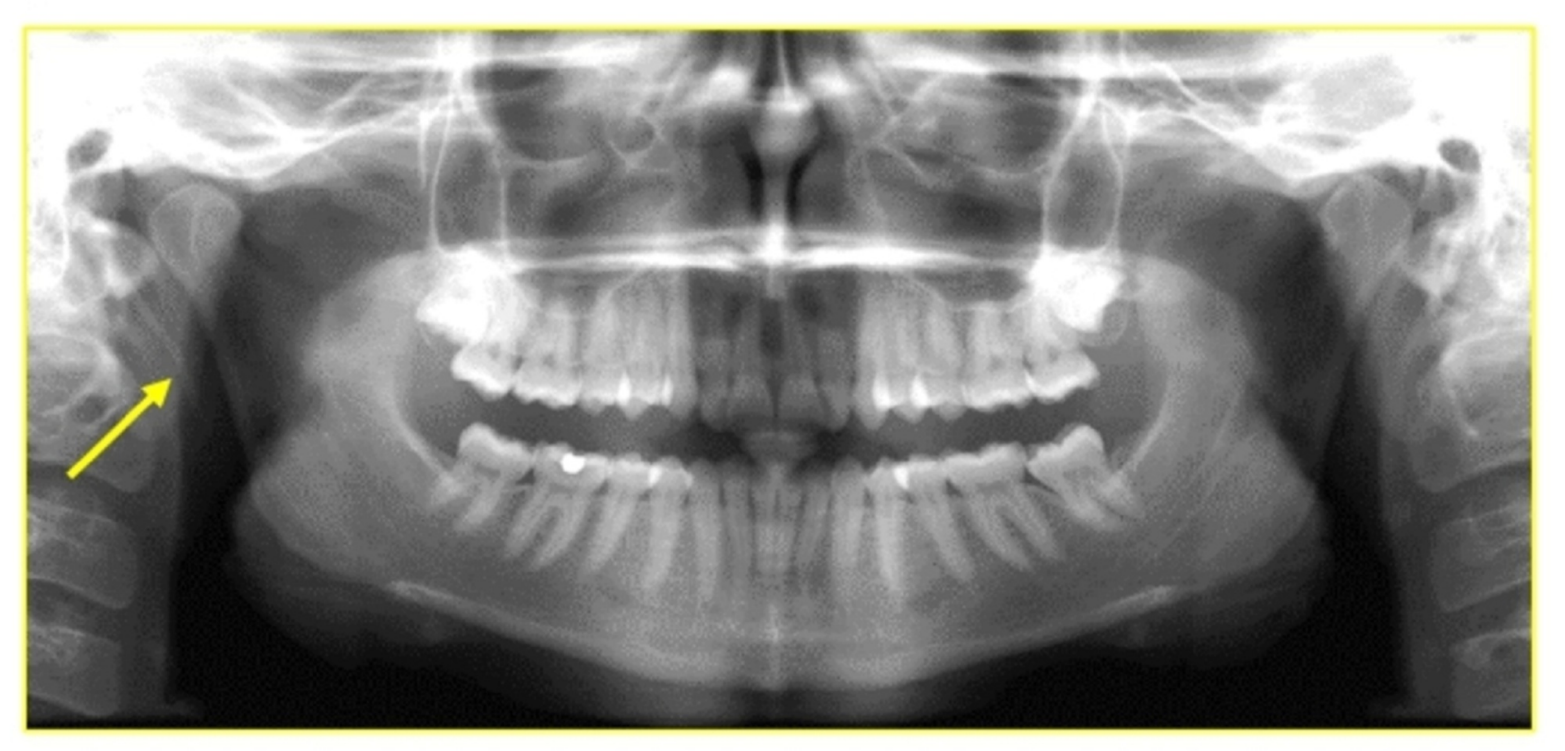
Panoramic radiograph showing the increased length of the styloid process (arrow) on the right side
